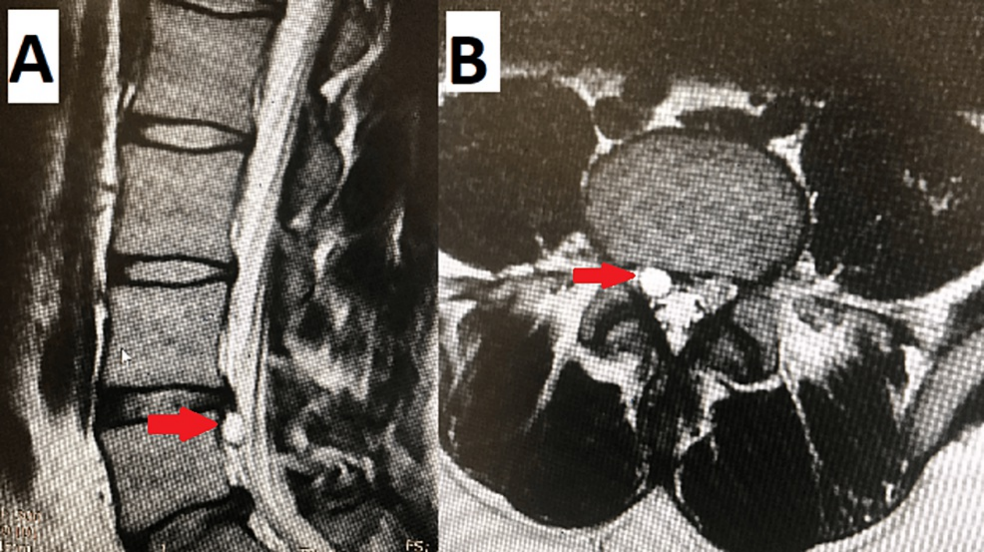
(A) Sagittal and (B) axial MRI with L4/5 posterior longitudinal ligament cyst (red arrow).
